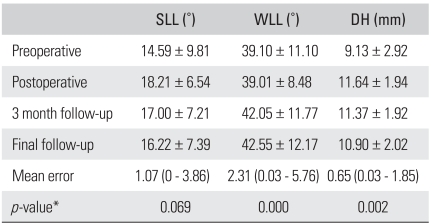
Changes in the Radiographic Parameters Affecting the Sagittal Balance (Mean ± SD)
